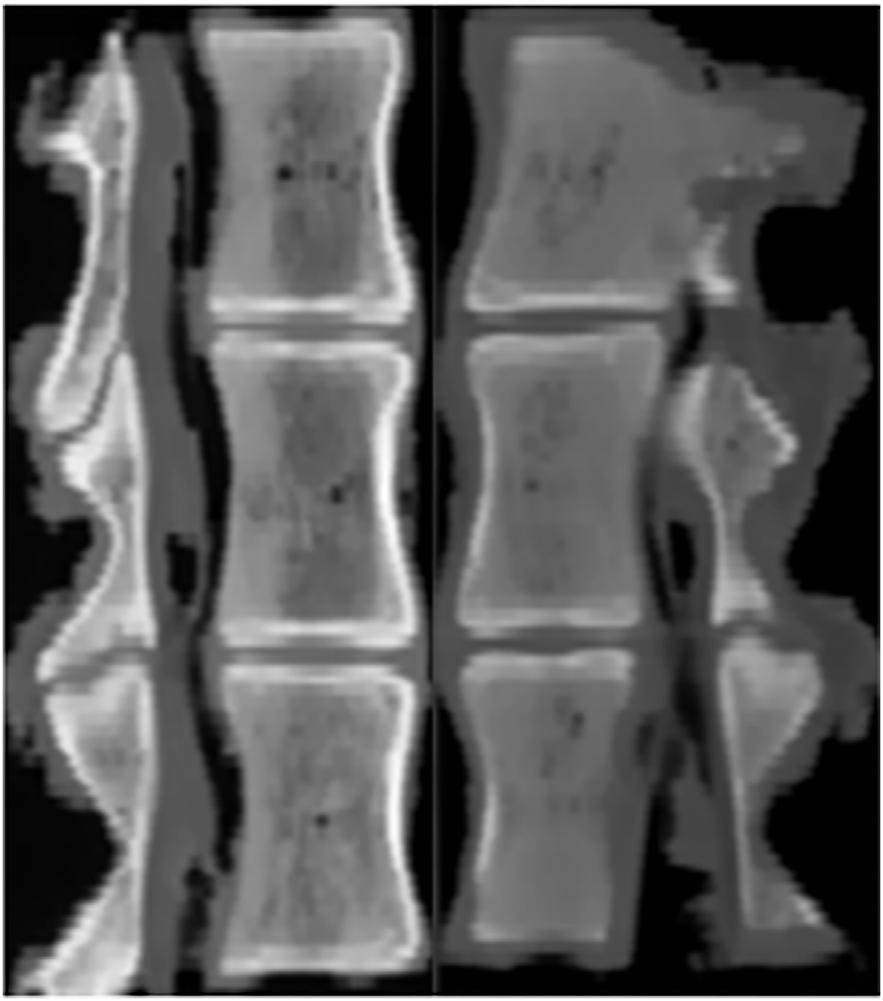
Sagittal reconstruction CT images before (left) and after (right) decalcification.
